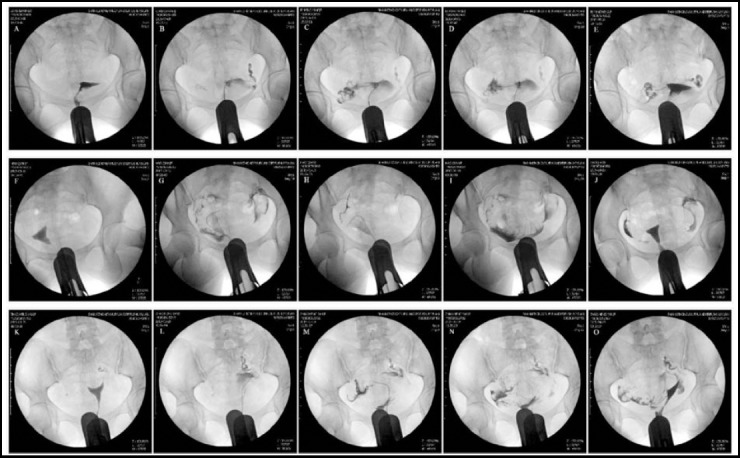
The process of the fallopian tube recanalization in multipara by ozone water perfusion. A, F, K: cervix, uterine cavity and salpingography showed bilateral fallopian tube obstruction; B, G, L: the guide wire was used to dredge the left fallopian tube.1-2ml contrast agent was injected to determine the unobstructed fallopian tube; C, H, M: the right fallopian tube was dredged using the guide wire. 1-2ml contrast agent was injected to determine the unobstructed fallopian tube. D, I, N: the uterine cavity and bilateral fallopian tubes were perfused using 20ml 40ug/ml ozone water. E, J, O: after the fallopian tube obstruction recanalization then the first menstrual cycle for 3-7 d, the uterus and bilateral fallopian tubes radiography was performed under the digital subtraction X ray machine. The result showed that obstruction of bilateral fallopian tubes were removed.
